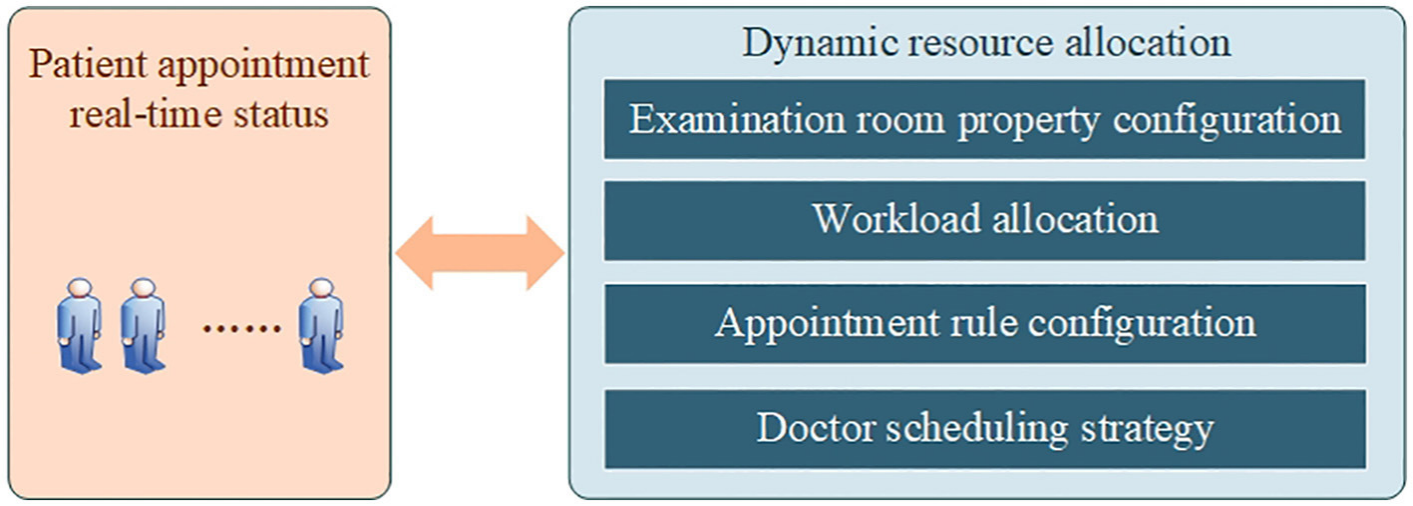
Real-time dynamic resource allocation strategy of the ultrasound appointment system.
